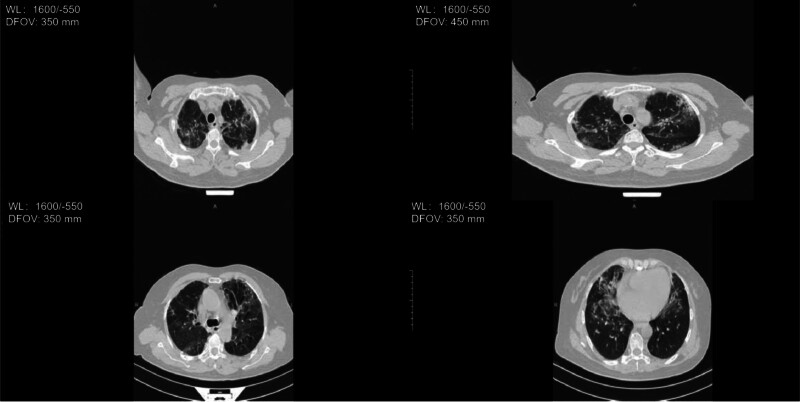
Chest CT on September 20, 2023, showed significant resolution of the bilateral patchy and strip-like high-density opacities compared with previous imaging. CT = computed tomography.
